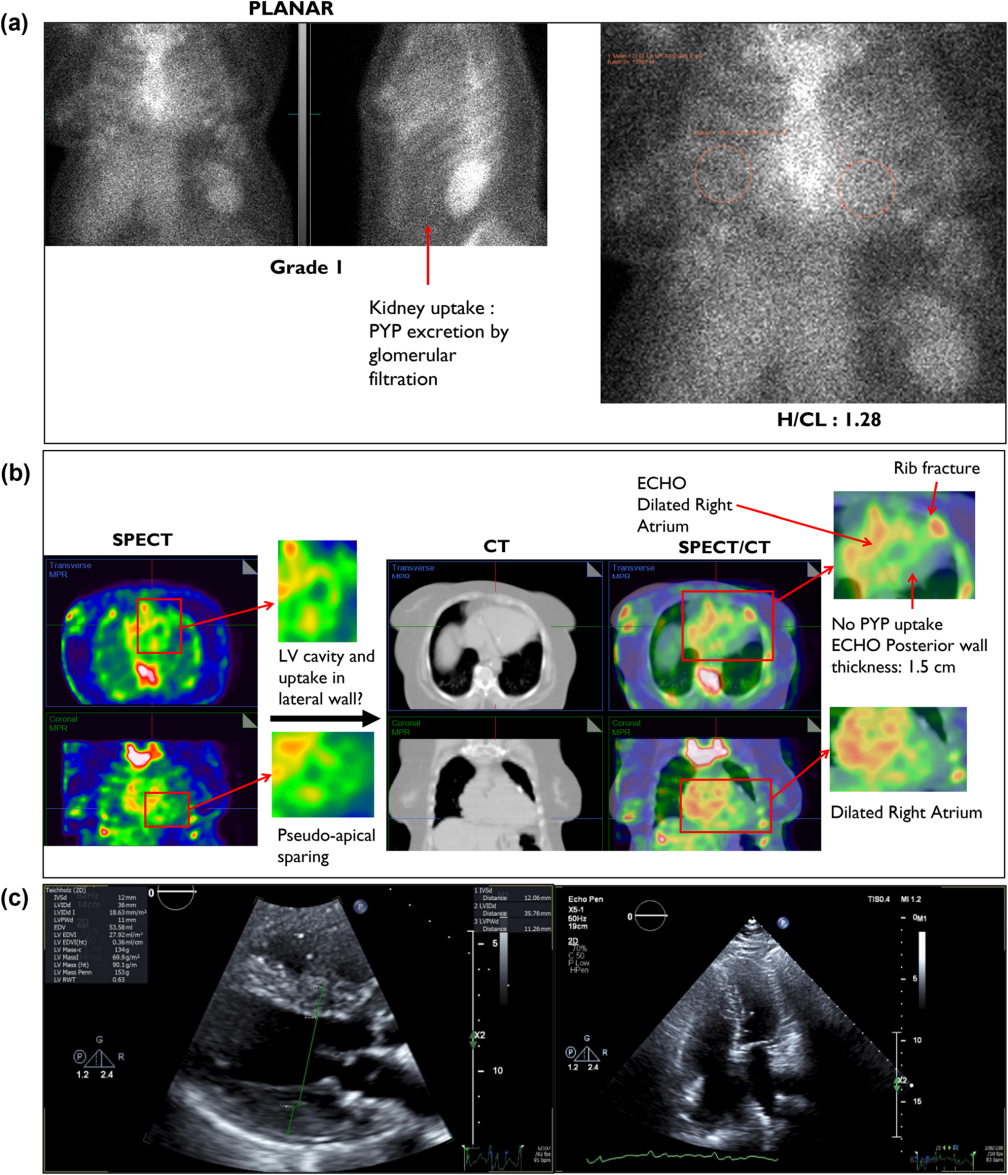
(A) Three-hour planar imaging showing Grade 1 tracer uptake and H/CL of 1.28; interpreted as “equivocal for ATTR amyloidosis”.^5^ Note the physiologic kidney uptake of PYP. (B) SPECT demonstrates empty looking LV cavity and a focal uptake in the rib on the right. There is also an *appearance* of apical sparing (pseudo-apical sparing). Note the rib fracture and enlarged RA and left atrium (LA). SPECT/CT demonstrates no PYP uptake in the LV myocardium, specifically in the thickened posterior wall. SPECT/CT reclassifies case from equivocal to “not suggestive.” (C) ECHO shows posterior wall thickness of 1.5 cm. Note dilated RA and LA
